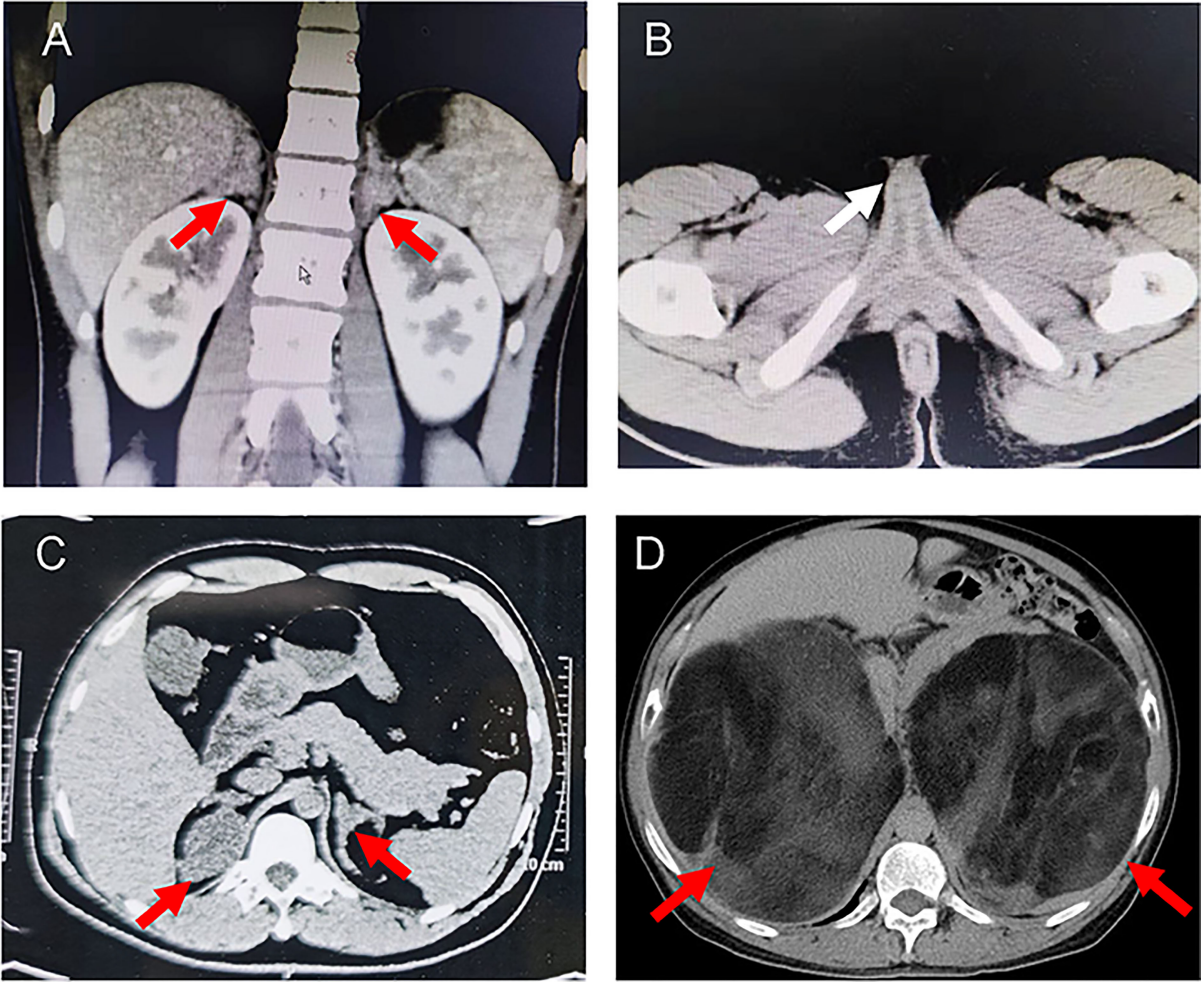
Representative computed tomography (CT) scans of the probands. (A) CT images showing bilateral adrenal hyperplasia in the proband 1. (B) Pelvic CT image of proband 1 indicated the cavernous structure in the perineum (white arrow). (C) CT images showing left adrenal hyperplasia and right adrenal adenomatous hyperplasia (4.3*3.7 cm) in the proband 2. (D) CT image showing giant bilateral adrenal masses (left: 20*25 cm; right: 30*40 cm) in the proband 3. The red arrows indicate the adrenal lesions.
